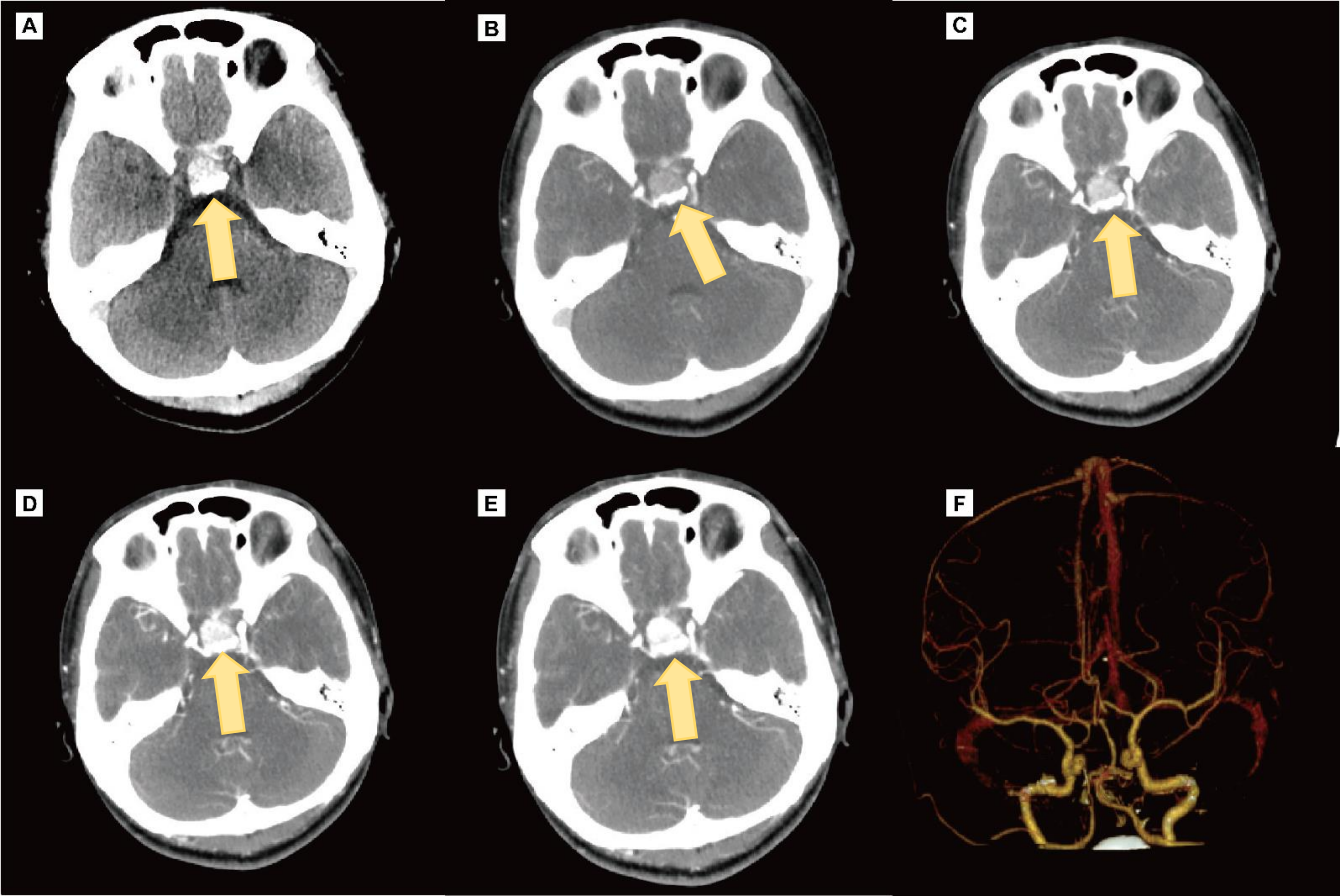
Preoperative Intracranial Artery CTA. (A) Coronal CT plain scan shows a round high-density area in the sellar region (as indicated by the arrow). (B, C, D, and E) Coronal CT-enhanced scans reveal enhancement within the lesion (as indicated by the arrow). (F) 3D reconstruction of computed tomography angiography (CTA) shows no abnormalities.
